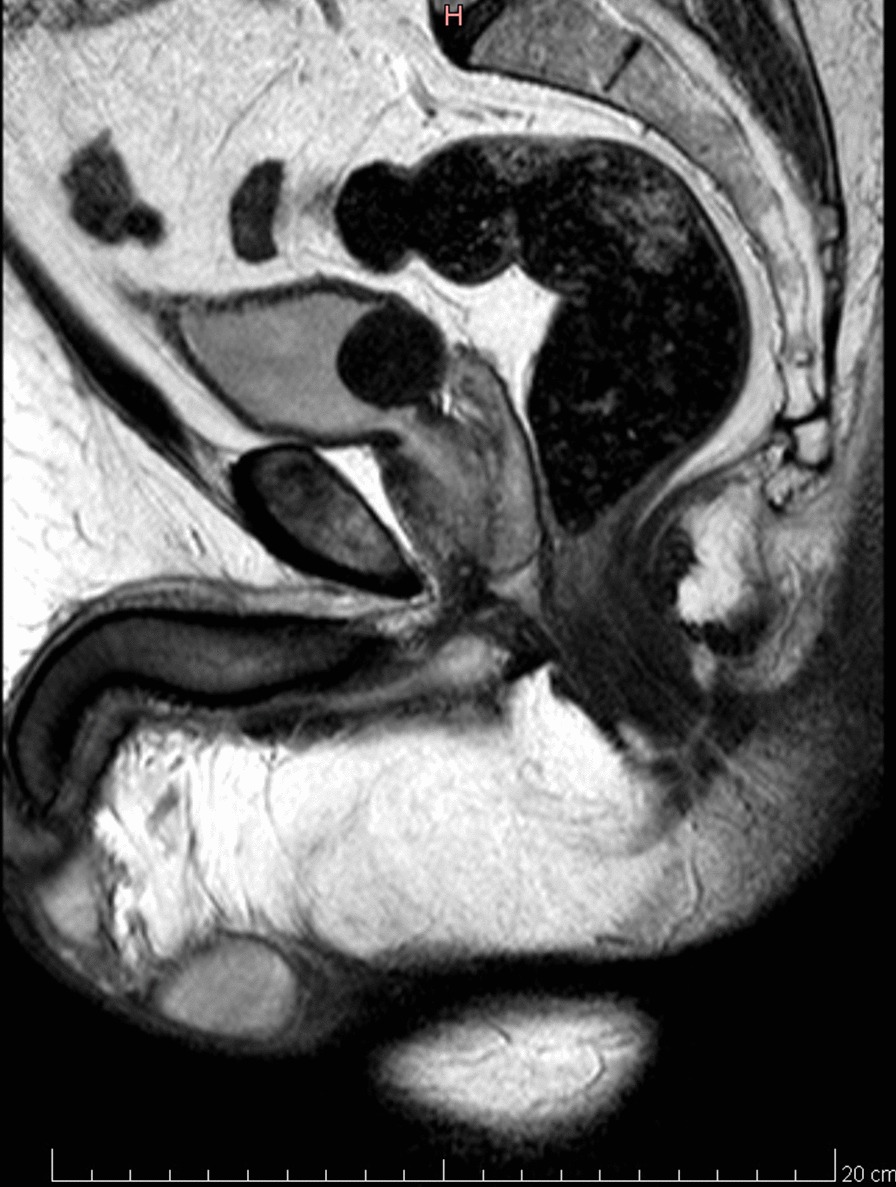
MRI sagittal view of leiomyoma
